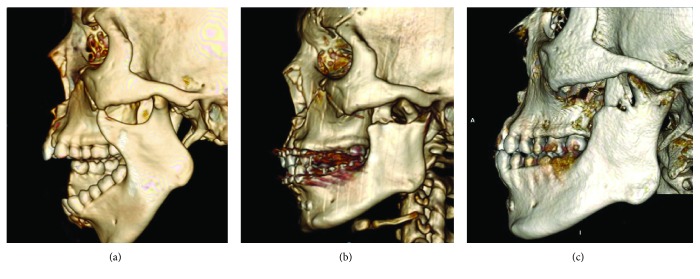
CT scan image of the left temporomandibular joint at (a) day 1 of trauma, (b) two days postsurgery, and (c) one year postsurgery.
